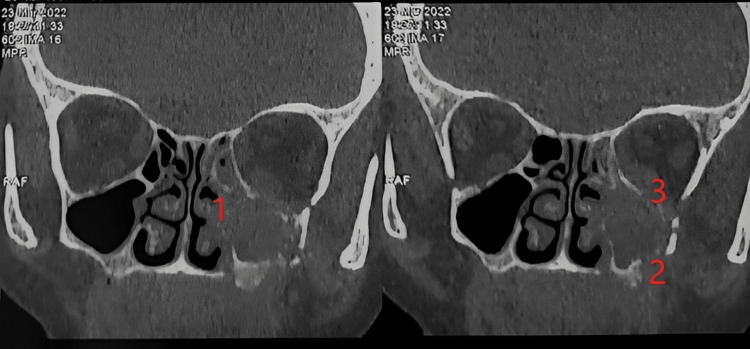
CT image of one of the mucormycosis patients, showing destruction of the medial wall (1), the anterolateral wall (2), and the roof (3) of the left maxillary sinus.
